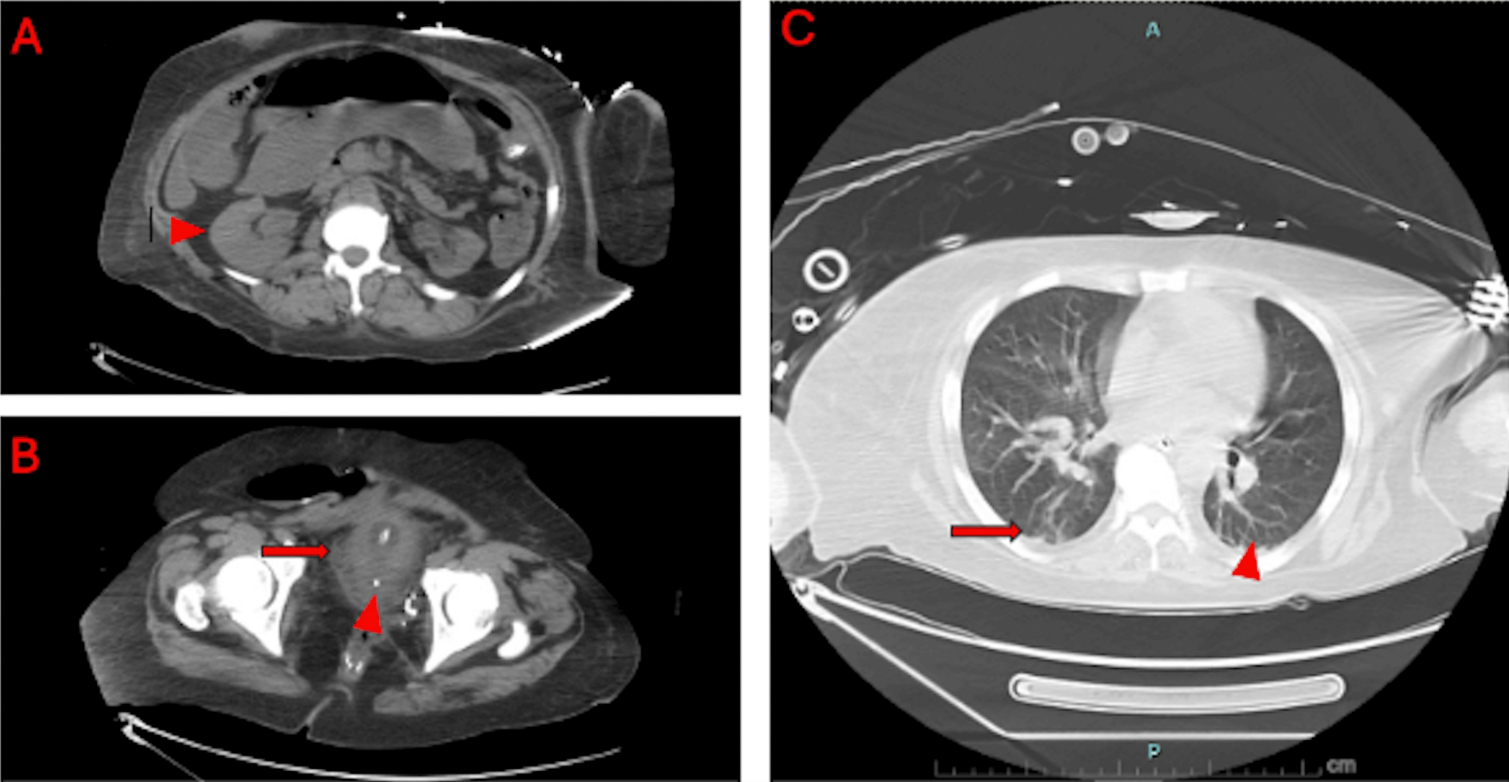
Axial CT scan of the abdomen and pelvis without contrast. (A) Left pyelitis (arrowhead). (B) Bladder calculi (arrowhead) and bladder fat stranding (arrow). (C) Lower lung opacities (arrow) and venous congestion (arrowhead).
